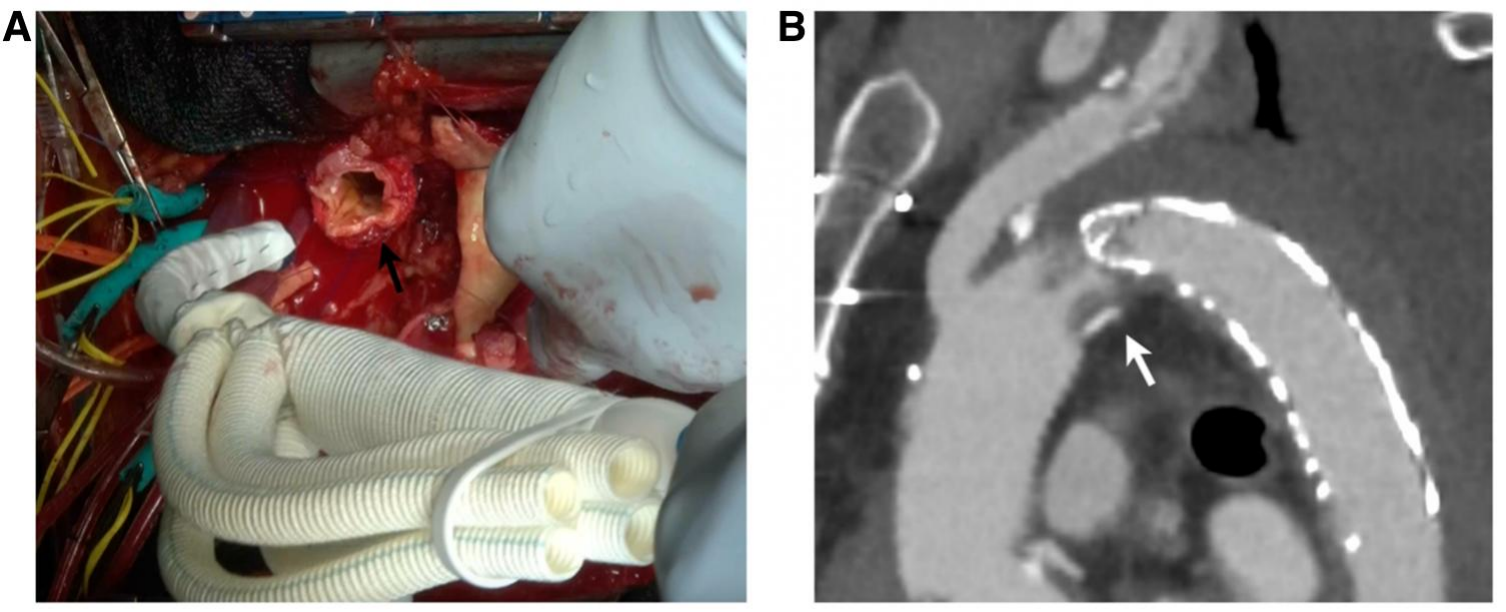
(A). Intraoperative images show small diameter of aortic arch (black arrow). (B) Postoperative CT angiography shows that the graft narrowed at the joint (white arrow).
